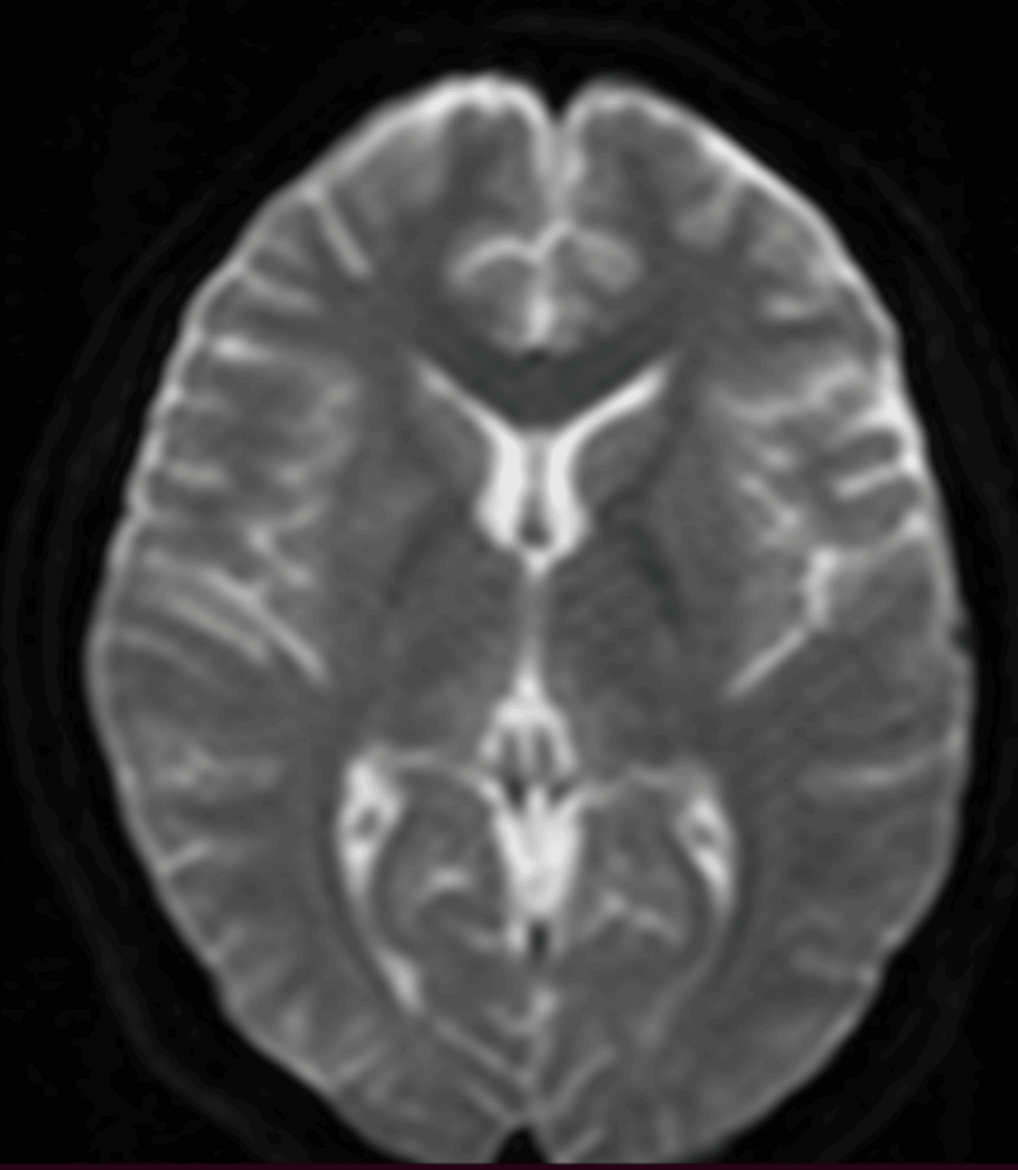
MRI of the brain, diffusion-weighted imaging (DWI), demonstrates no restricted diffusion
